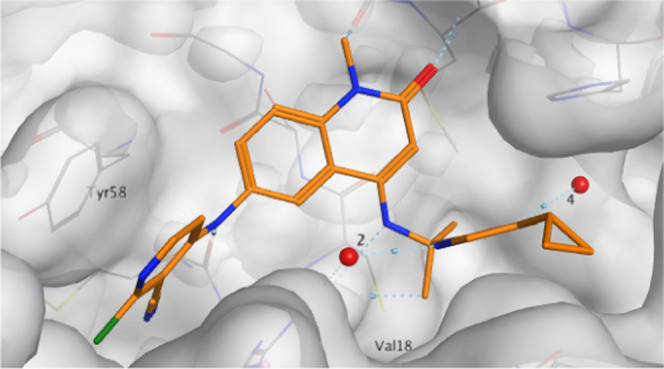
X-ray structure of 25 (PDB: 7OKD, resolution 1.94
